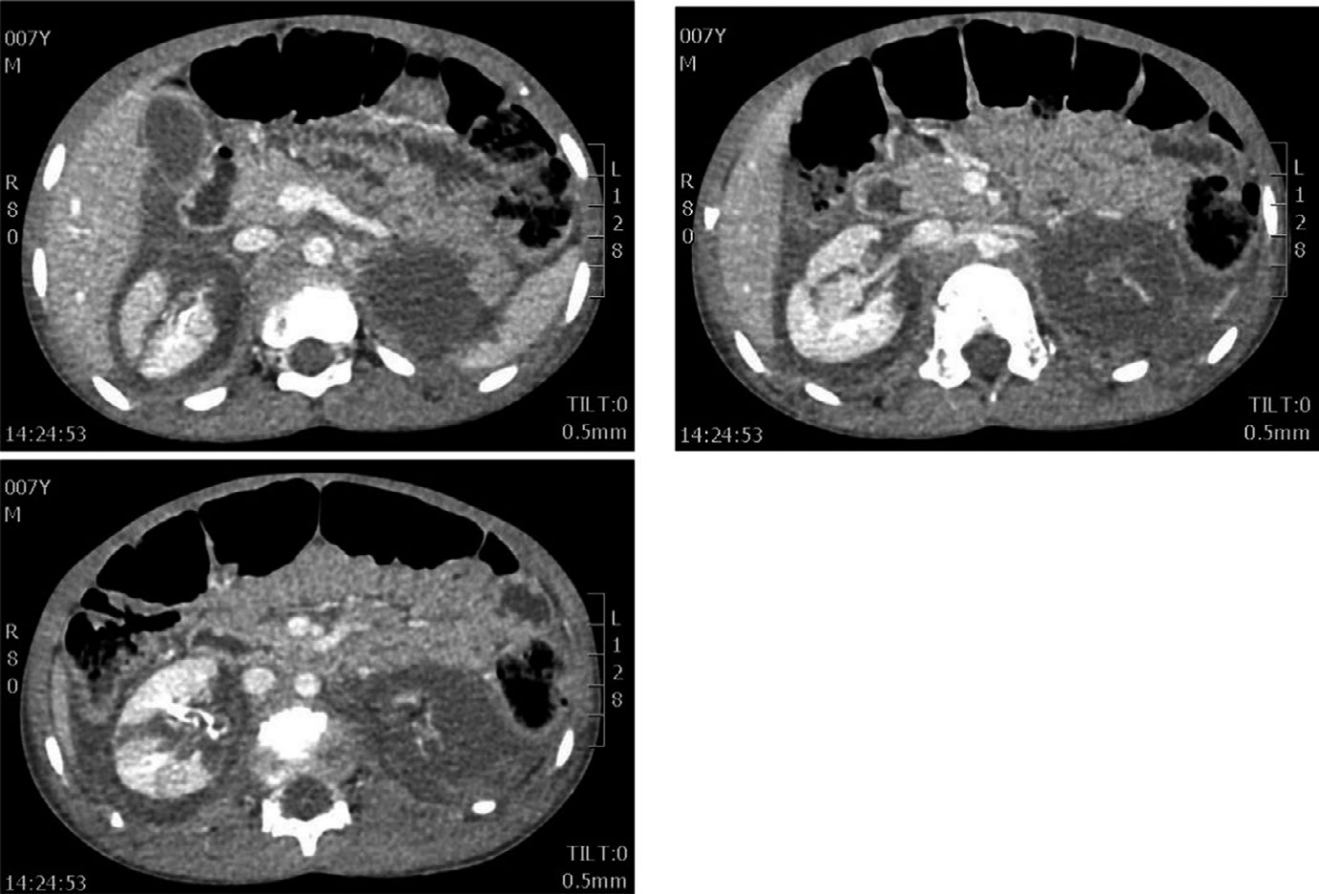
Contrast CT scan images showing grade 4 renal injury in the right kidney with uptake of contrast. The left kidney has a grade 5 renal injury with avulsion of the vascular pedicle with no uptake of contrast
